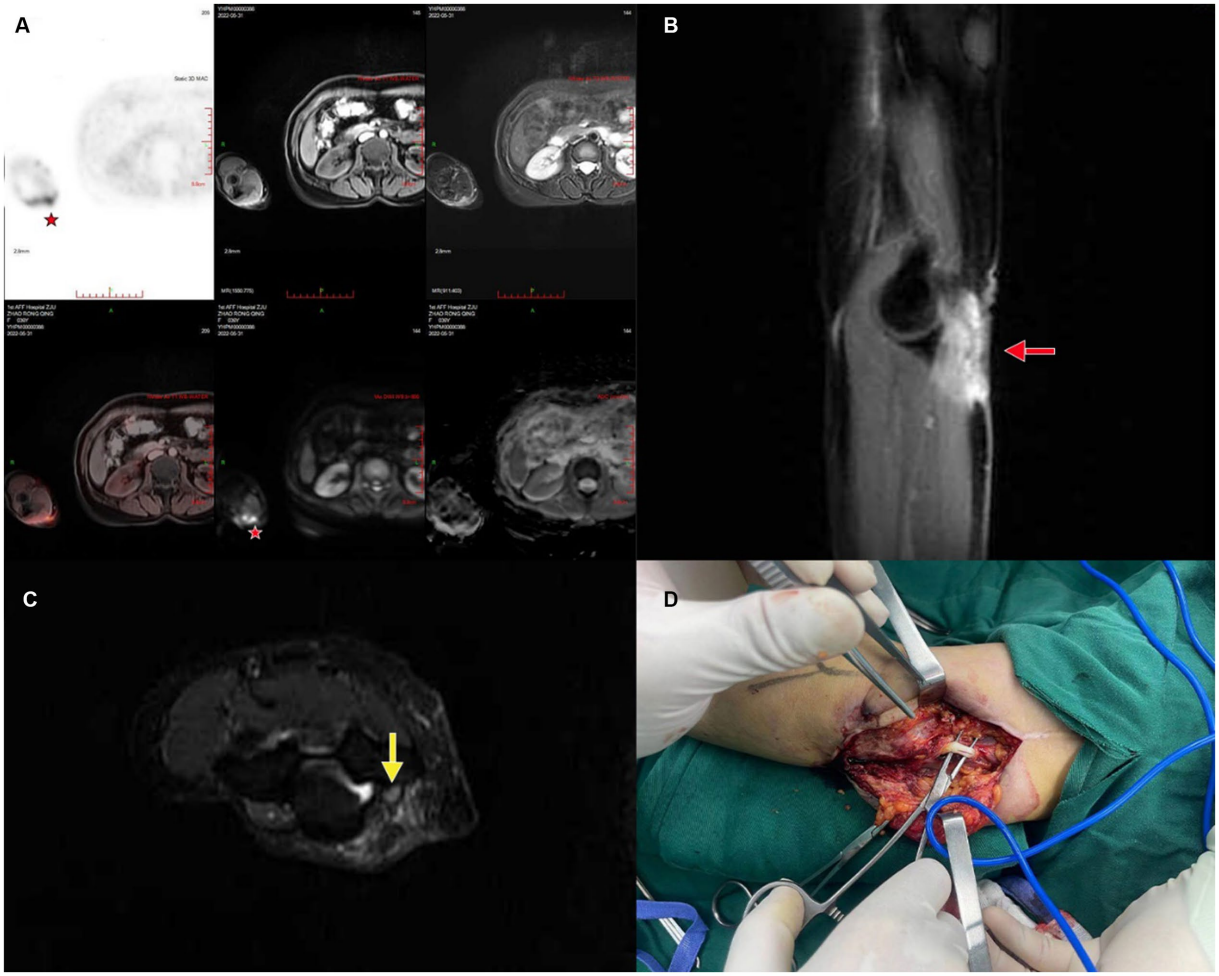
A case of patient suffering initially from allodynia and later a mass on the right elbow, which was pathologically confirmed as epithelioid sarcoma with ulnar nerve injury. (A) PET/CT showing the affected area; (B) coronal plane of the mass adjacent to ulnar nerve, red arrow marks the mass; (C) sagittal plane of the mass and the ulnar nerve, yellow arrow marks the swelling of the ulnar nerve; (D) intraoperative image of mass excision and nerve preservation.
